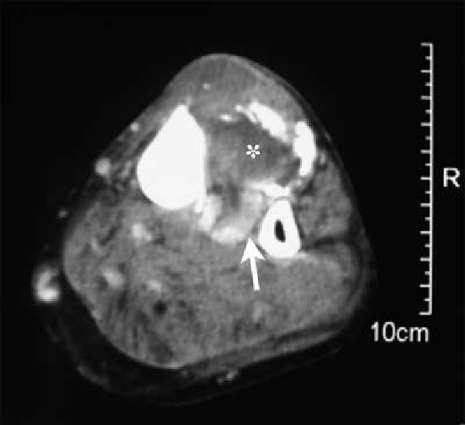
Postcontrast CT showed a 4×6 cm sized mass with central low signal density (asterisk) and peripheral high density, suggesting fluid and peripheral calcification. The space between the tibia and fibula was also enhanced (arrow).
